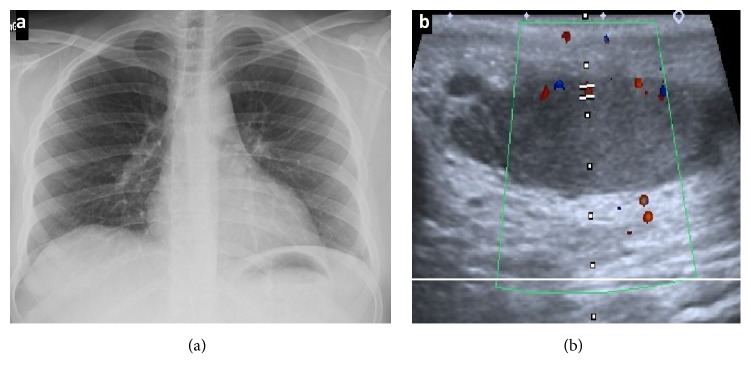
Chest X-ray. (a) There are no consolidations in the lung parenchyma or pleural effusion. US Testicular Doppler. (b) Testicle and left epididymis of normal size and vascularization.
